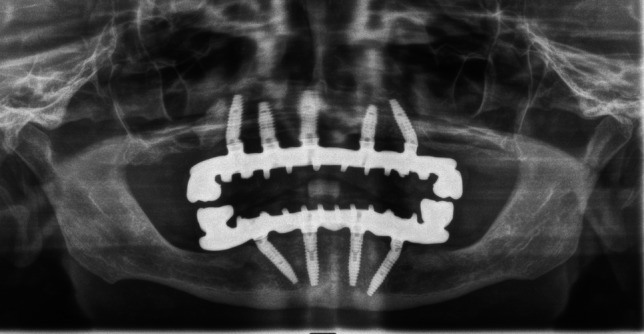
Orthopantomography of bimaxillary ISFP including zirkonium molars (Zr-ISFP)
